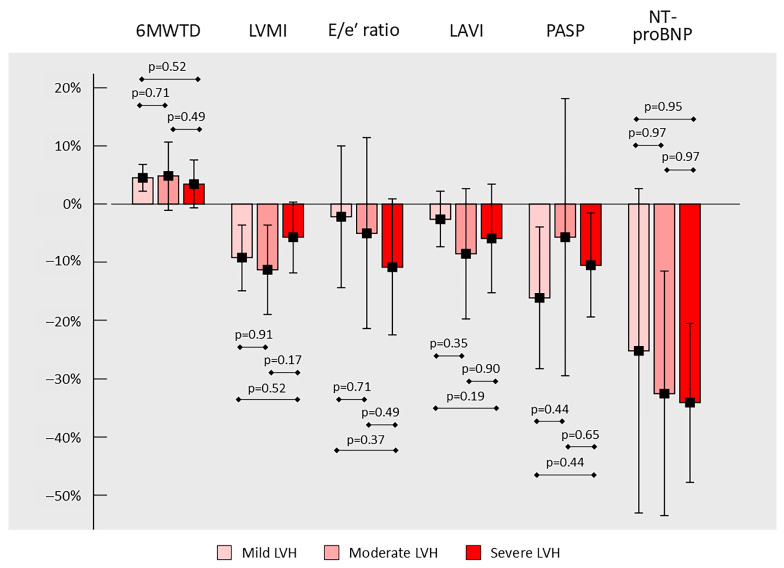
The effect of Sac/Val on key clinical and echocardiographic parameters in HFpEF patients depending on the severity of LVH. The data are presented as percentage changes from baseline. Bars and squares indicate mean changes, while markers (error bars) indicate 95% confidence intervals. E, early inflow velocity; e′, averaged annulus relaxation velocity; LAVI, left atrial volume index; LVH, left ventricular hypertrophy; LVMI, left ventricular mass index; NT-proBNP, N-terminal pro-brain natriuretic peptide; PASP, pulmonary artery systolic pressure; Sac/Val, sacubitril/valsartan; 6MWTD, 6-min walk test distance.
